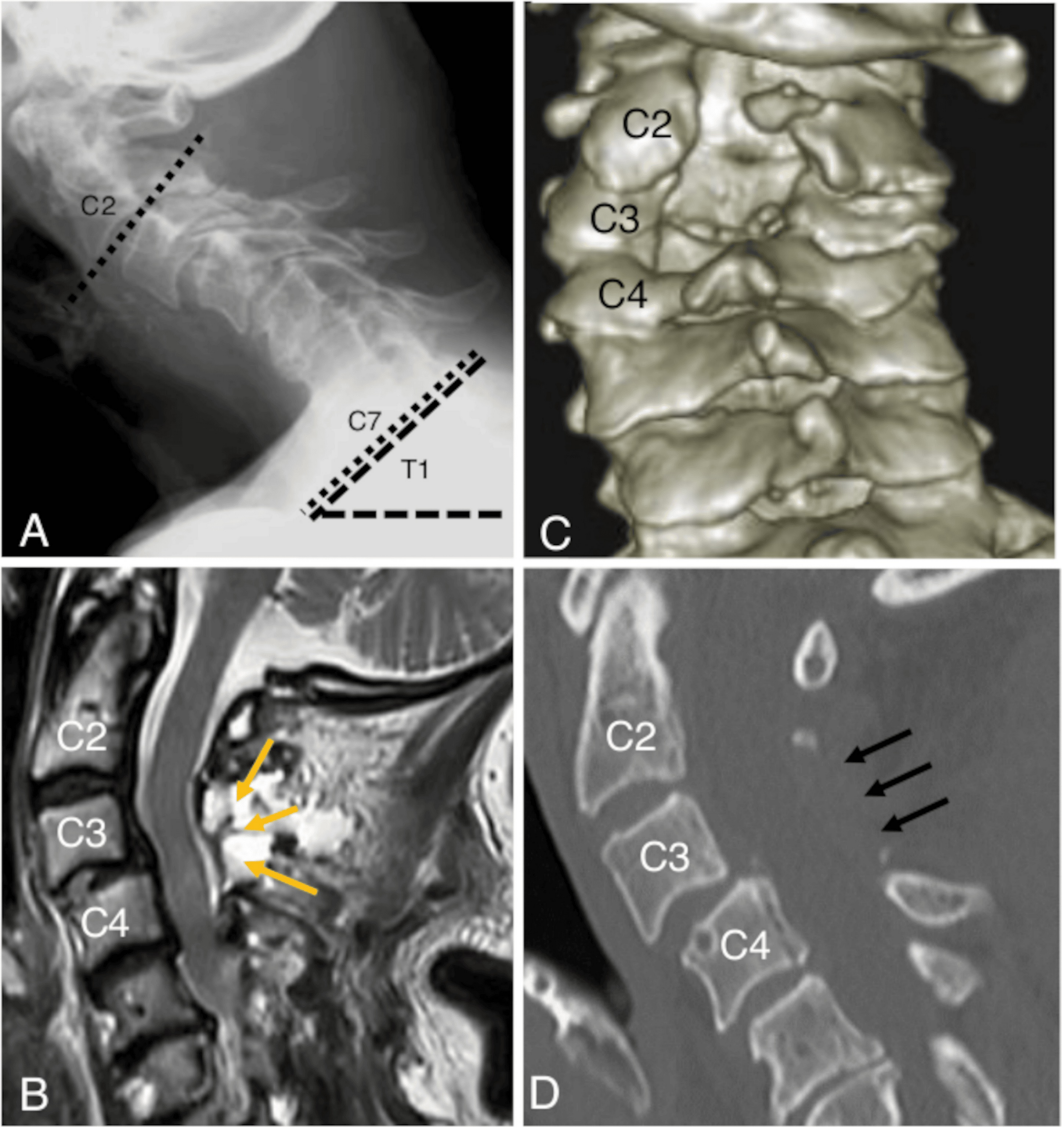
Postoperative images (A) Nine months after surgery, an X-ray showed the C2-C7 Cobb angle (dotted lines) and T1 slope (dashed lines) as -5° and 42°, respectively. (B) A follow-up magnetic resonance image revealed decompression of the spinal cord from C2 to C4 (yellow arrows). (C, D) Postoperative computed tomography images showed hemilaminectomy from C2 to C3 and partial laminectomy of C4 (black arrows).
